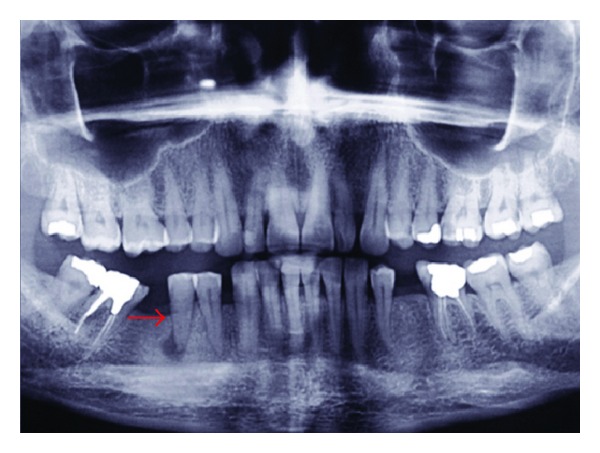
Preoperative radiograph; the pulp space of the right second mandibular premolar has been seriously obliterated and periapical radiolucency is observed at the apex of the tooth.
